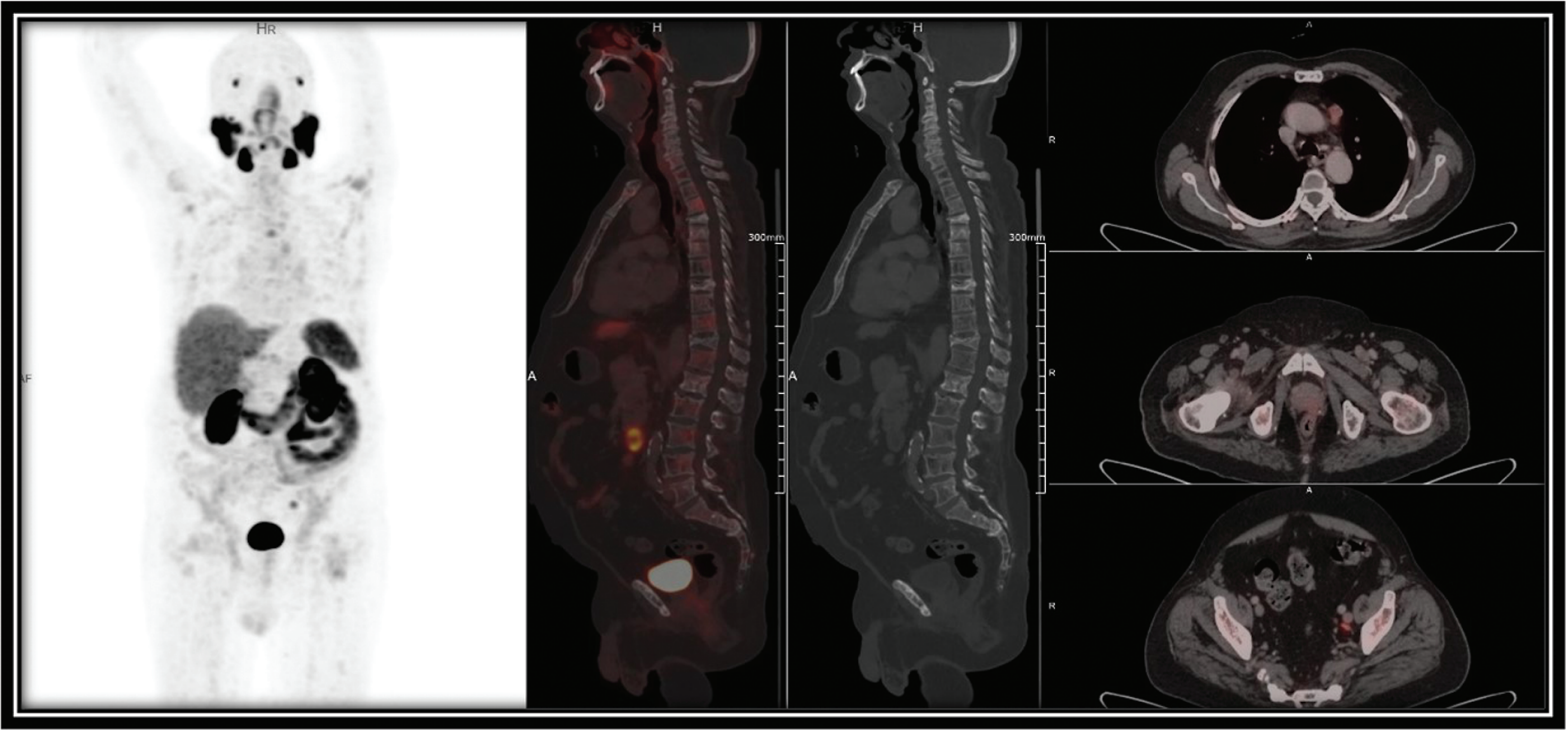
Prostate-specific membrane antigen positron emission tomography/computed tomography ([
